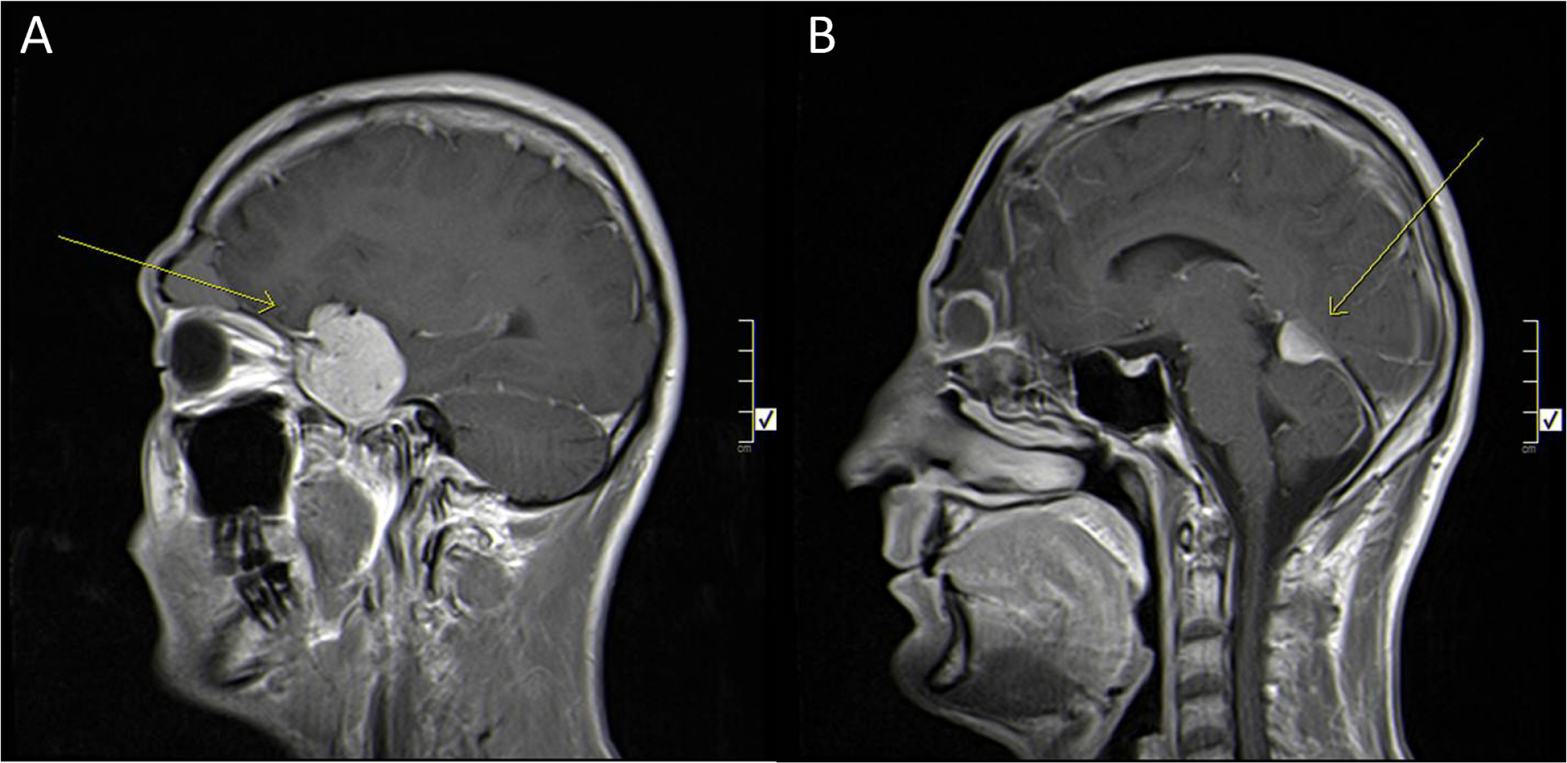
Brain MRI. Sagittal view. Post-contrast T1 weighted image. Showing a. recurrent mass in the infratentorial region, b. recurrent mass in the left temporal lobe
